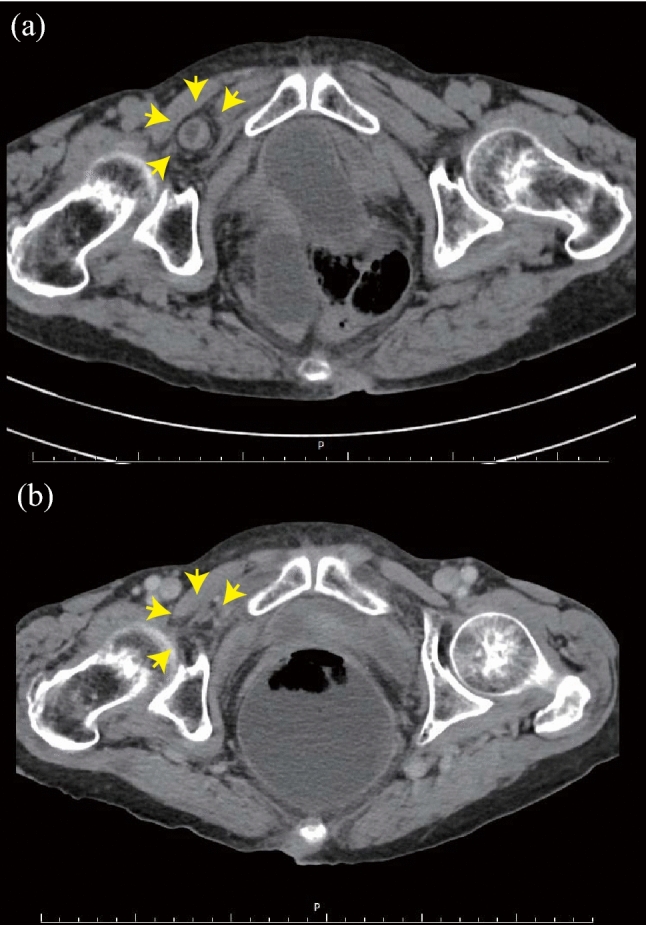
(a) Computed tomography showed an incarcerated small intestine (arrows) before manual reduction. (b) After reduction, the incarcerated small intestine disappeared (arrows).
